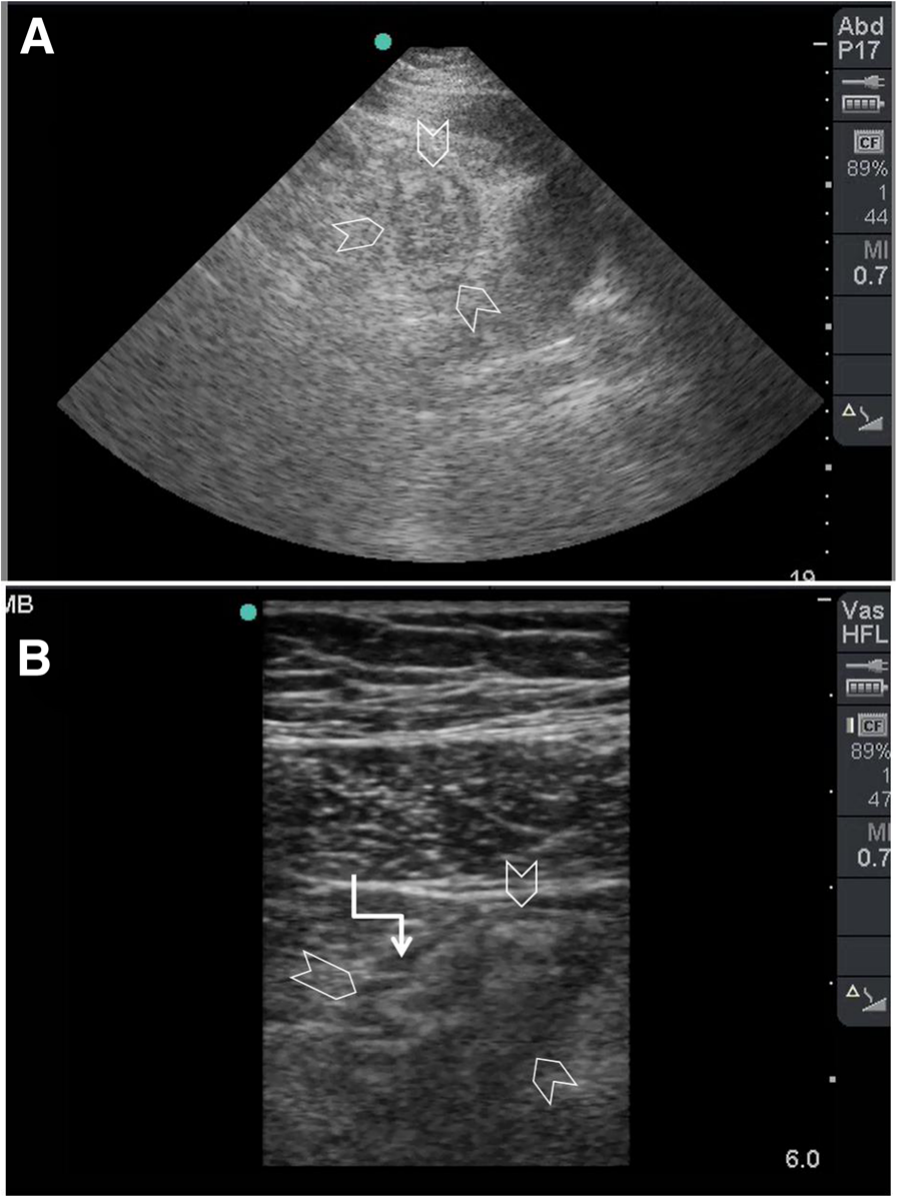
A 32-year-old man presented with left lower quadrant (LLQ) abdominal pain of 5 days duration. Abdominal examination revealed a tender mass in the LLQ. The patient had leukocytosis and raised CRP. POCUS study (a) using a portable ultrasound machine and a small print convex array probe with a frequency of 3–5 MHz has shown a rounded mass (arrowheads). A more detailed image (b) using a high-frequency linear probe showed that the mass (arrowheads) had a rounded folded edge giving it the classical *saw-tooth pattern.* The hypoechoic area represents the muscularis propria (arrow)
